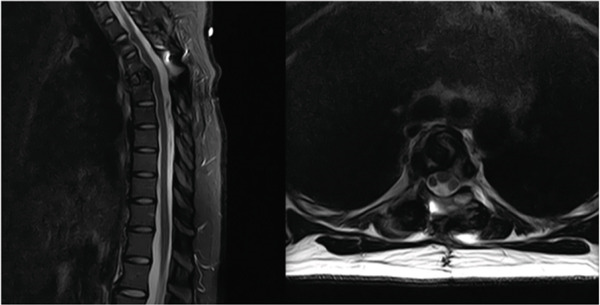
Eight‐month follow‐up MRI demonstrating decompression after T5 corpectomy with T3–T7 posterior fusion.
